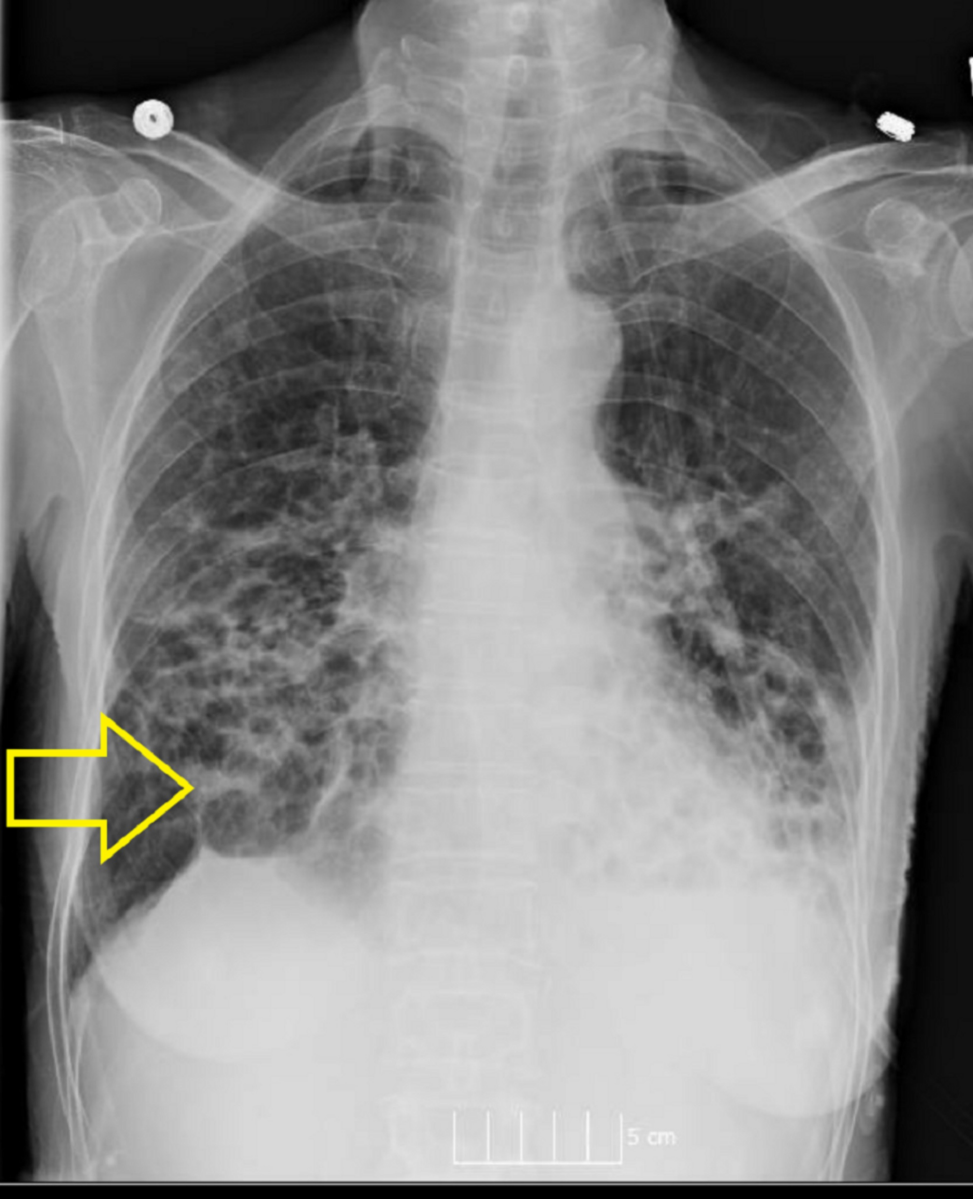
Chest radiography showing extensive cystic changes and bronchiectasis.
